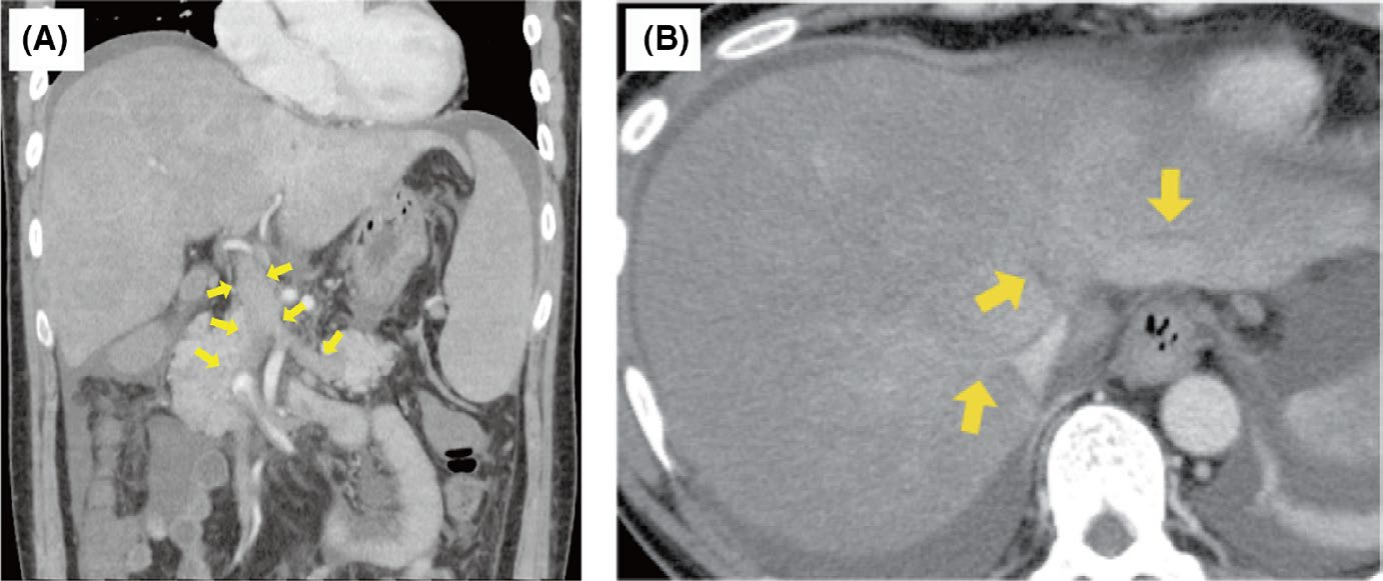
Coronal contrast‐enhanced computed tomography in the portal venous phase shows decreased attenuation in the portal vein and splenic vein without any signs of cavernous transformation. Yellow arrows indicate thrombosis (A). Axial contrast‐enhanced computed tomography in the portal venous phase shows decreased attenuating thrombus in the three main hepatic veins (arrows), compression of inferior vena cava, enlargement of the liver, and presence of ascites (B)
